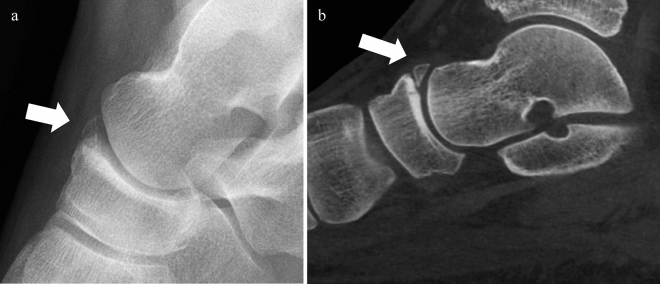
Example of false positive: a 44-year-old patient with an acute trauma of the ankle. The XR on a lateral view concluded to an acute talonavicular avulsion as no clear corticalization of the bone fragment was seen. CBCT on b lateral plane of the same patient: fragment related to a chronic avulsion of talonavicular ligament without acute pattern (white arrow)
